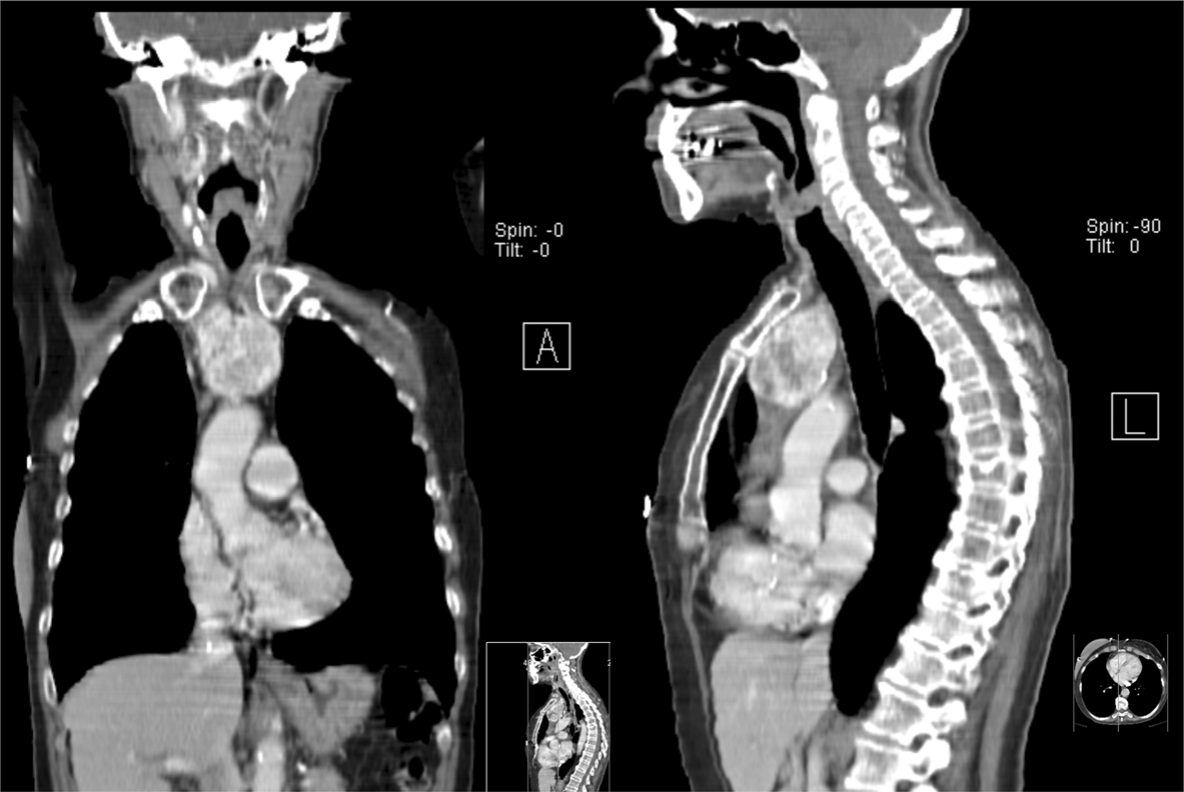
Neck and thorax computed tomography scan – coronal (*left*) and sagittal sections (*right*)
